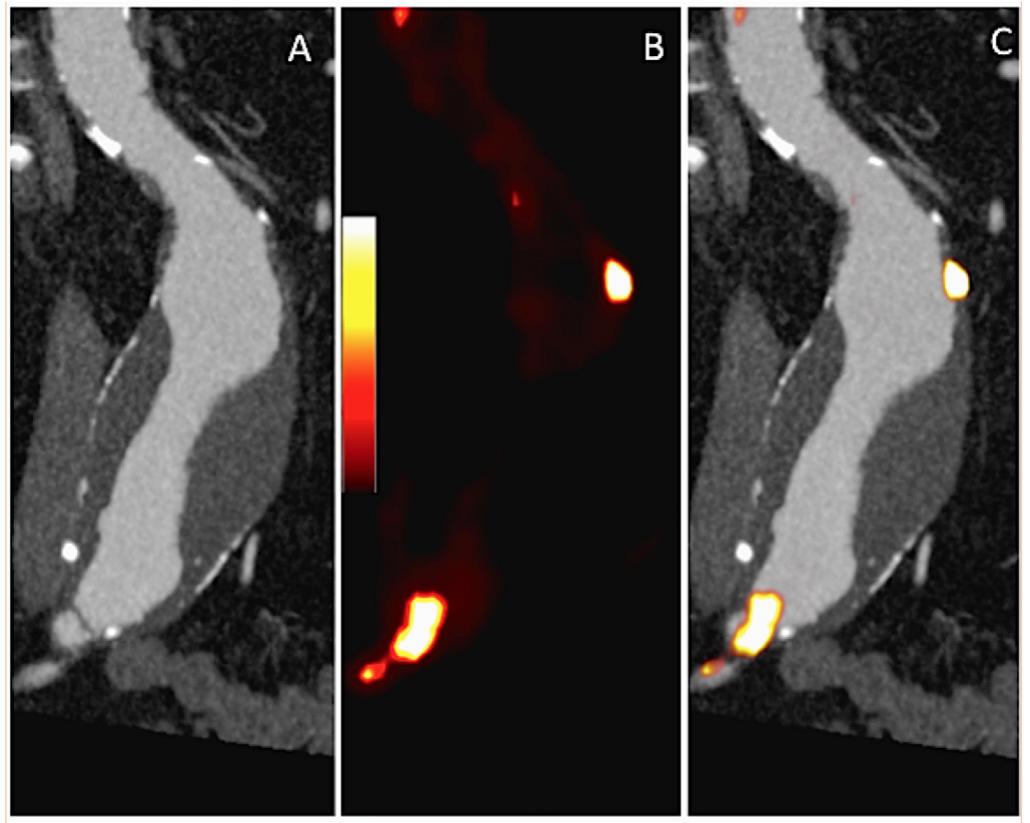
Coronal contrast CT, 18F-FDG positron emission tomography (PET) and fused images from a subject with a 4.5 cm aneurysm demonstrating focal 18F-FDG uptake within the aneurysmal wall (golden colour on the PET images). Note also the presence of intraluminal thrombus and calcification.
